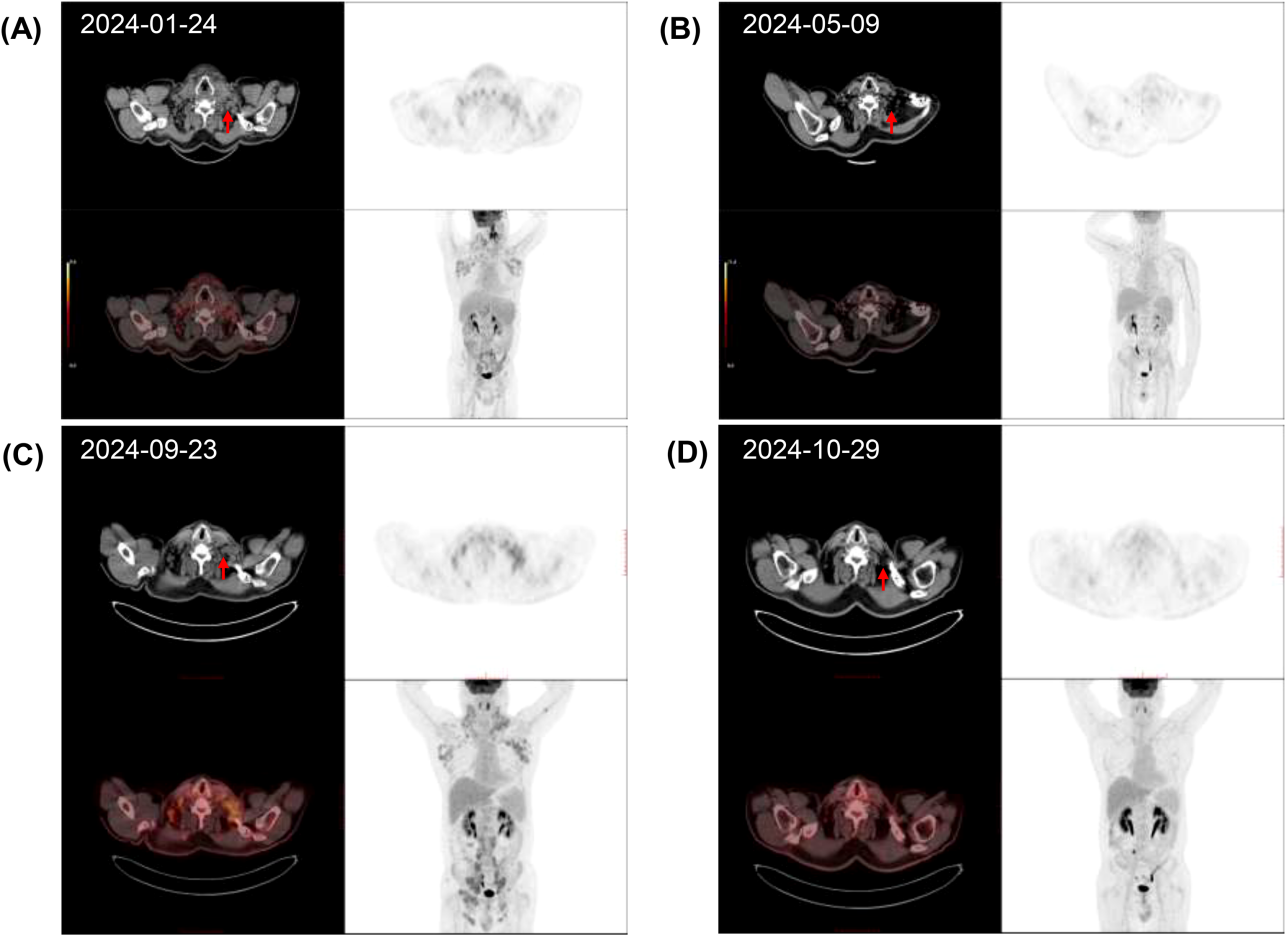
Dynamic evaluation of enlarged lymph nodes using PET-CT and CT. Enlargement and reduction of lymph nodes at initial diagnosis (A), PR (B), PD (C), and CR (D) by PET-CT evaluation. The red arrows indicate the lymph nodes.
